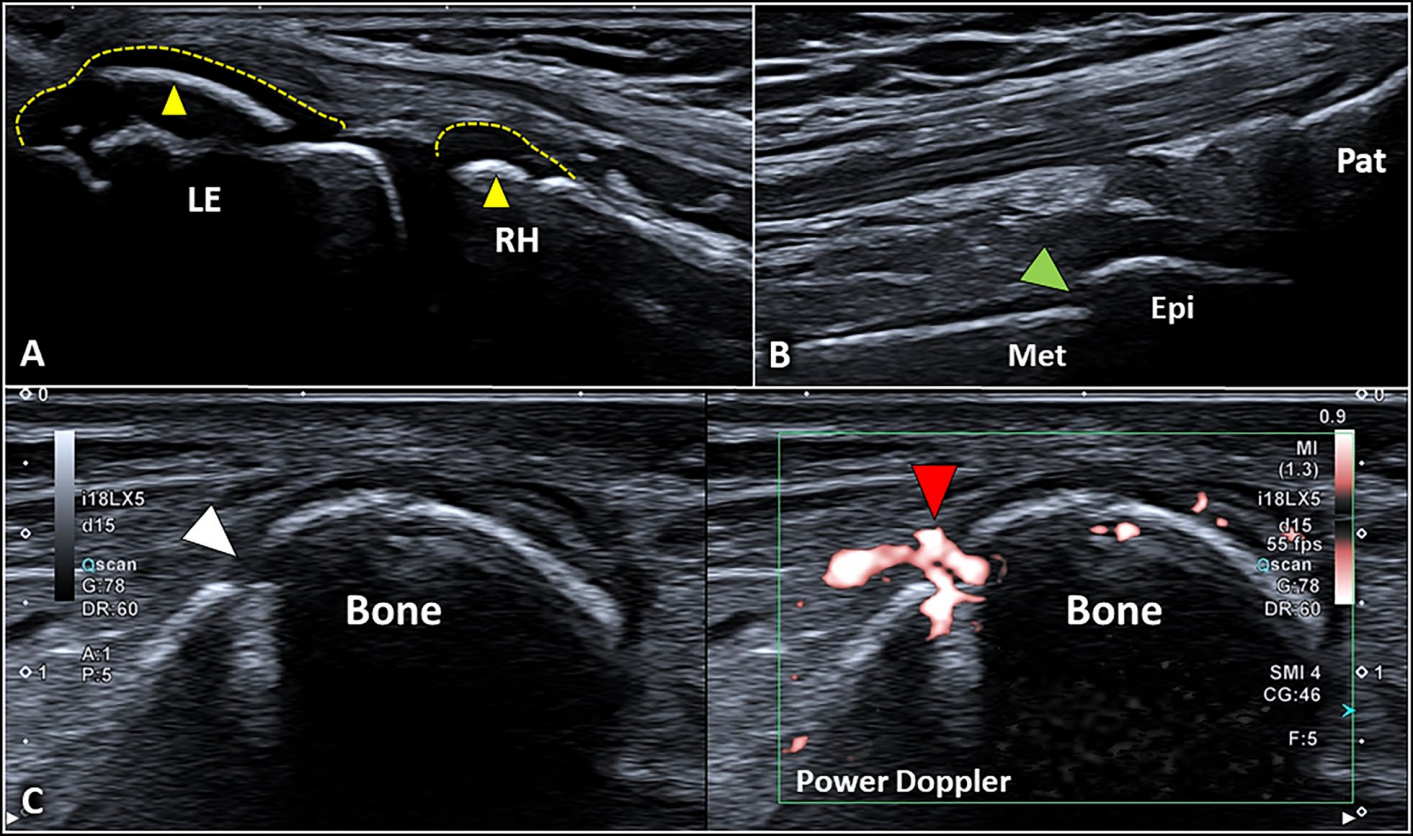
Normal sonographic findings of the bone tissue. A longitudinal view of the lateral elbow in a young volunteer clearly shows the cartilaginous epiphysis (*yellow dotted lines*) of the radial head (*RH*) and lateral epicondyle (*LE*); of note, the hyperechoic lines (*yellow arrowheads*) within the hyaline cartilage are the epiphyseal ossification centers (A). Likewise, a longitudinal scan of the suprapatellar region in the knee shows the physis (*green arrowhead*) in between the metaphysis (*Met*) and epiphysis (*Epi*) of the distal femur in a child (B). Importantly, focal interruption of the (hyperechoic) cortical bone can be related to the presence of nutritional foramina (*white arrowhead*) crossed by feeding vessels (*red arrowhead*) (C). *Pat* patella
